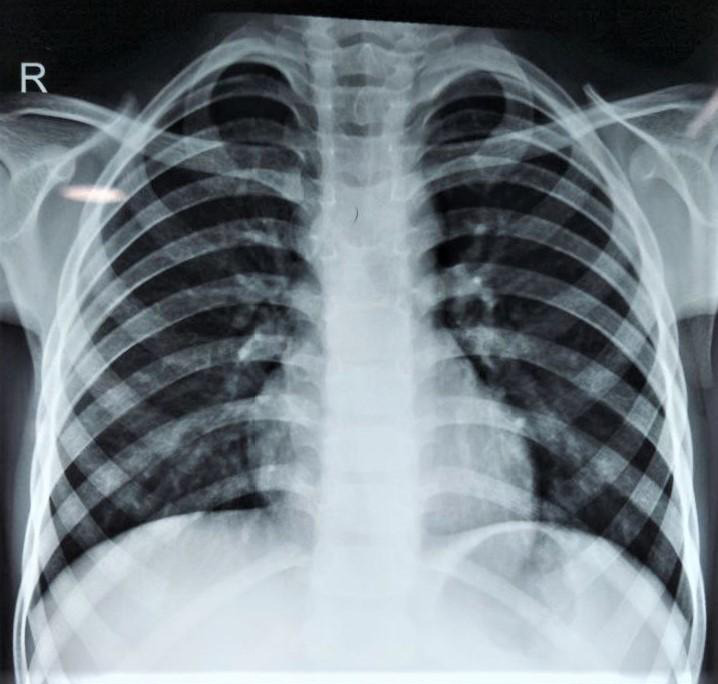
Chest radiograph (Normal study).
